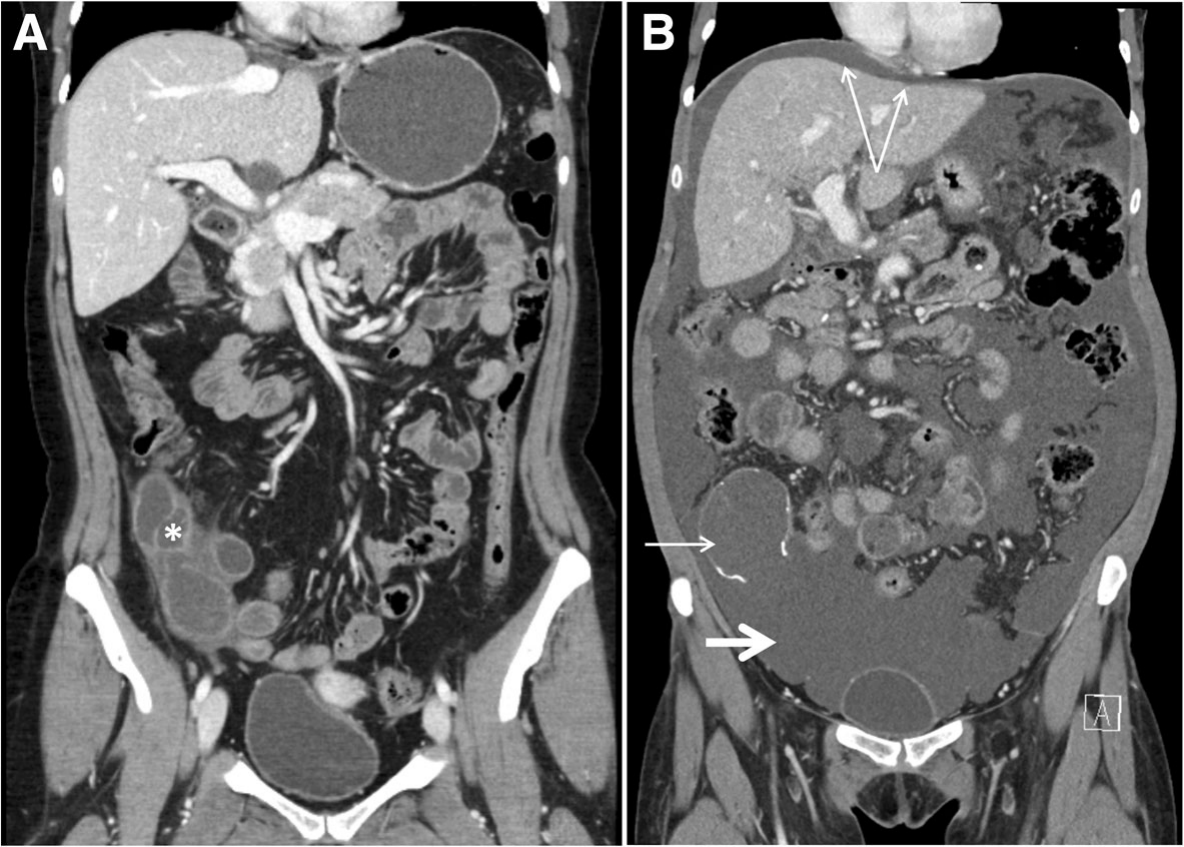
Development of pseudomyxoma peritonei (PMP). CT scans with coronal view before (a) and after (b) development of PMP. Mucinous cystadenoma of the appendix (asterisk) in a. Typical features of PMP (b): ruptured calcified appendix (short slim arrow), massive mucinous ascites (short thick arrow), central displacement of the small bowel, and visceral scalloping of the liver (double arrow)
